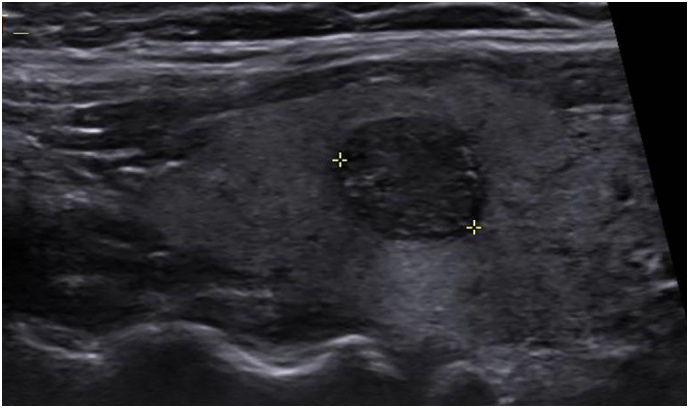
Ultrasound of the neck demonstrates a solid mass in the right lobe of the thyroid, demarcated between the two yellow cross hairs.
